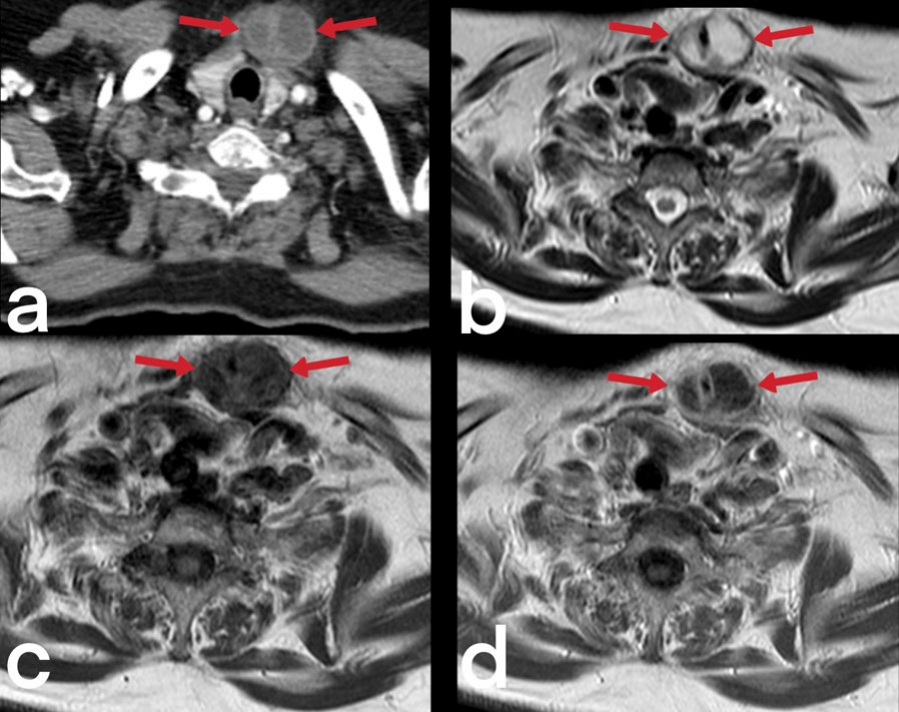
Axial contrast-enhanced CT (a), axial T2 weighted (b), pre (c), and post-contrast T1 (d) weighted images of a 69-year-old female. Biloculated abscess formation is seen in the anterior left paramedian region of the neck, which shows peripheral enhancement
